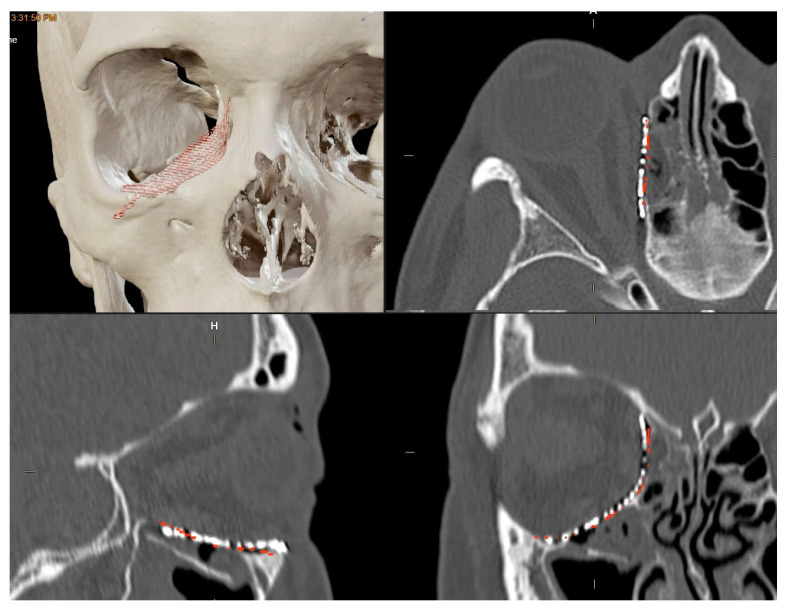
The overlaying of postoperative CT scans onto preoperative planning images.
